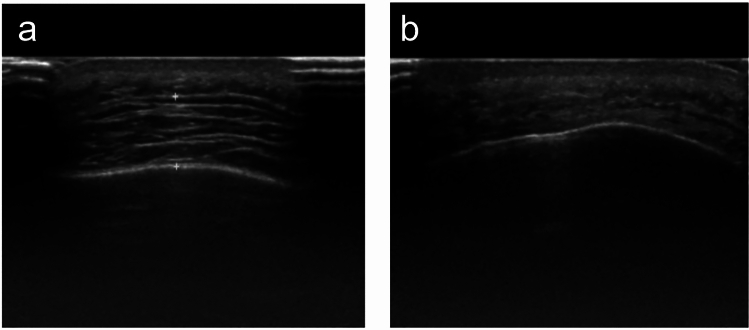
The images above show the ultrasound scans of a 45-year-old male patient with a lipoma on the forehead, before surgery (left) and 3 months post-surgery (right)
